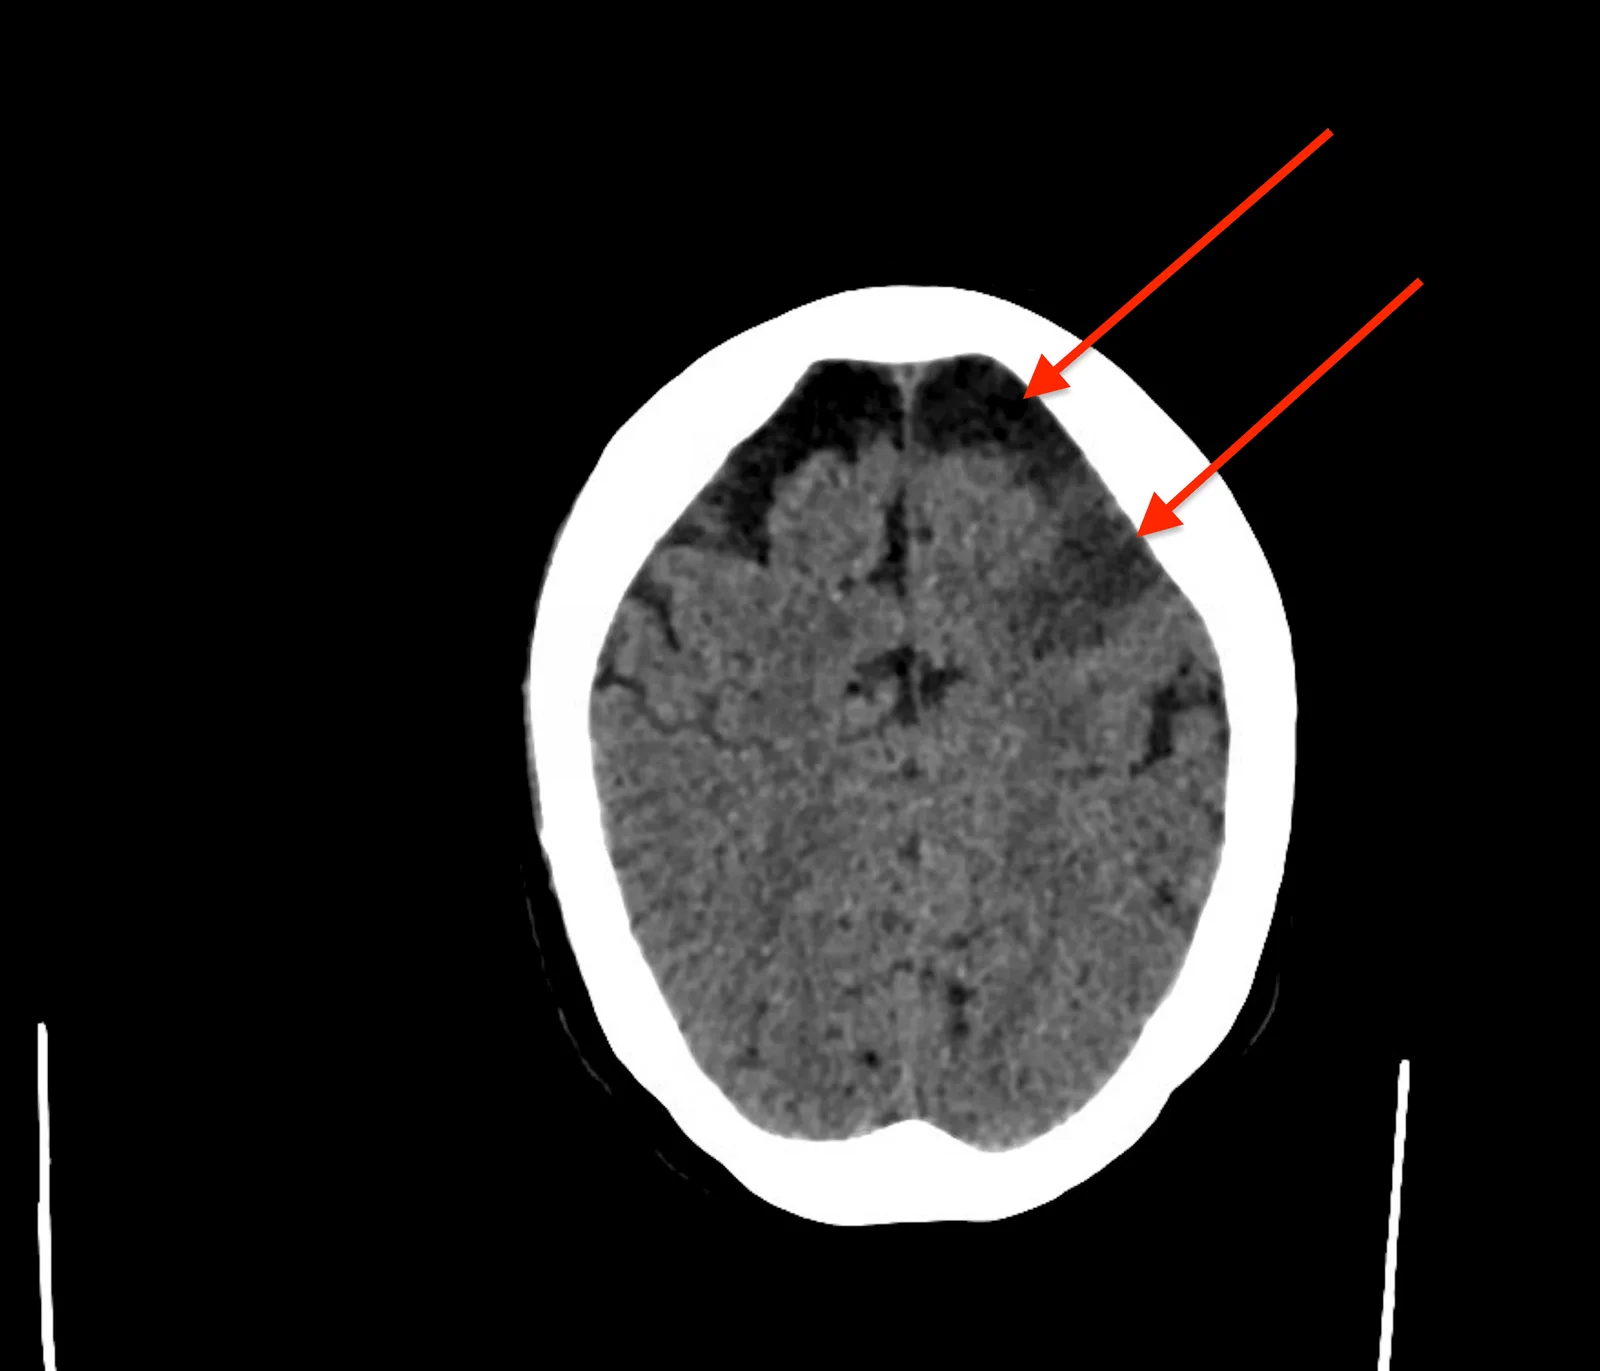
Non-contrast computed tomography (CT) head demonstrating septic embolic cerebral lesions Axial non-contrast CT image demonstrating wedge-shaped cortical and subcortical hypodensity involving the left frontal lobe (upper red arrow) with mild adjacent mass effect (lower red arrow), raising suspicion for septic embolic infarction. Subsequent MRI confirmed ring-enhancing cerebral abscesses.
